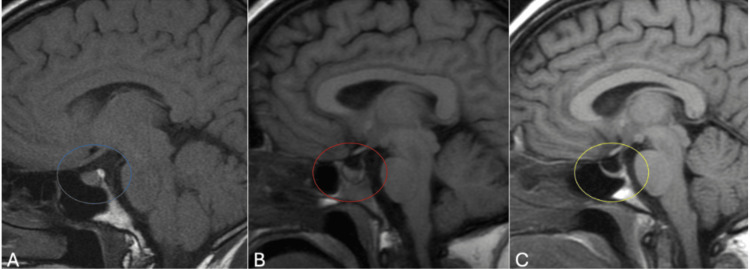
Partial vs complete empty sella on MRI. A. normal sella turcica (blue circle); B. partially empty sella turcica (red circle); C. complete empty sella turcica (yellow circle) (Taken from a free source published under Creative Commons License [28]). MRI: magnetic resonance imaging.
